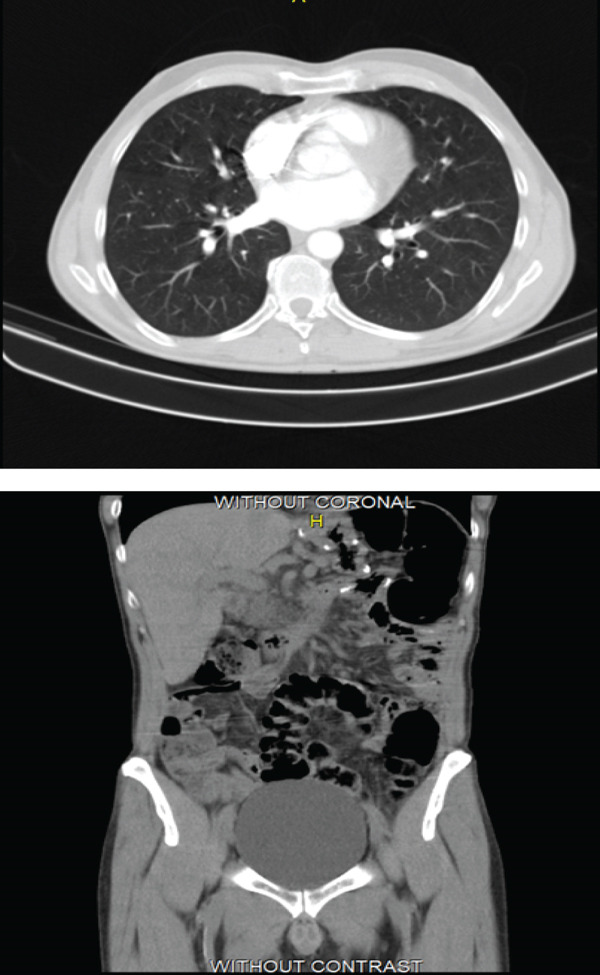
CT after abdominal TAP showing no abnormal radiological findings.
